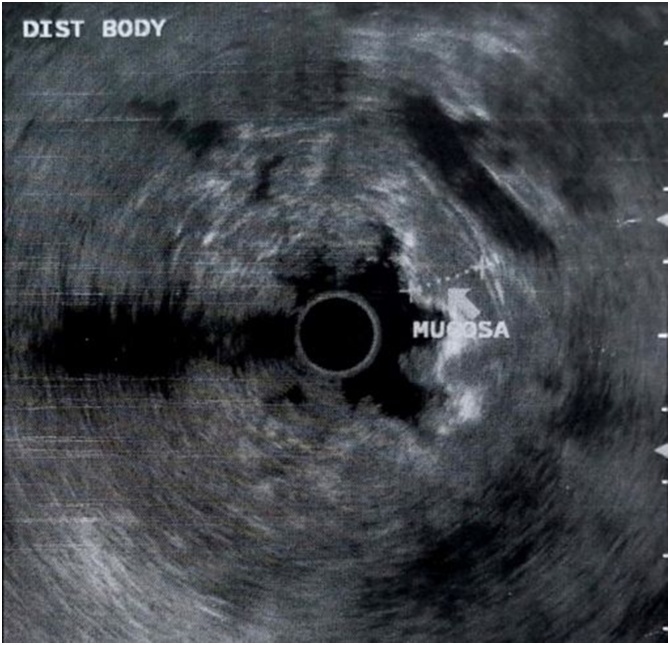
Endoscopic ultrasound from the distal body of the stomach.
